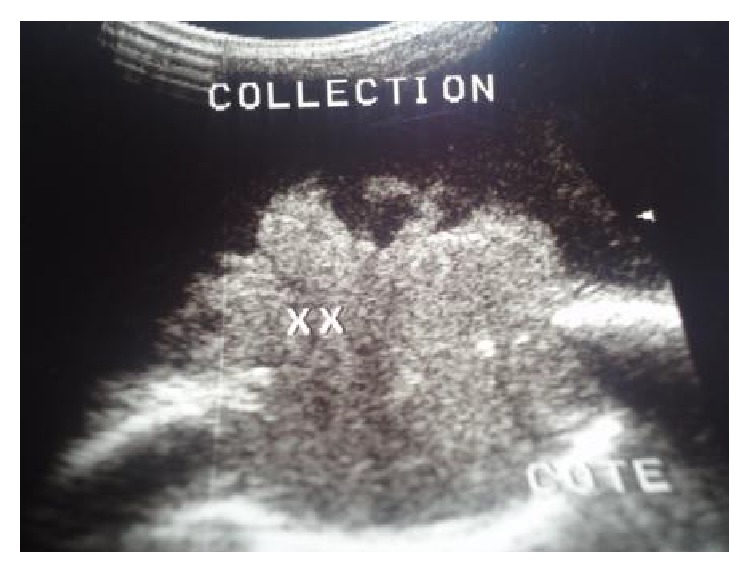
Ultrasonography showing hypoechoic lesion with dense internal echoes and destruction of rib.
